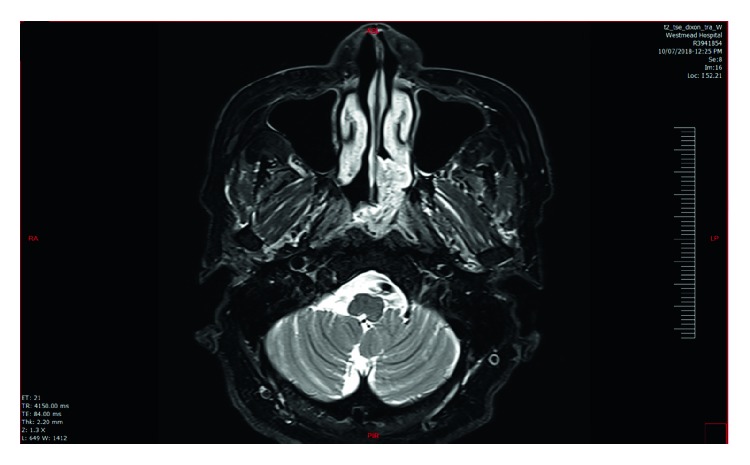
T2 MRI brain showing the mass with cerebriform appearance with extension into right posterior nasal space.
